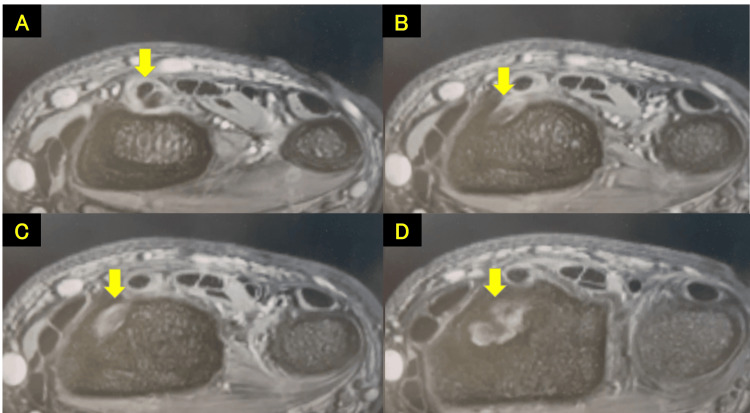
Magnetic resonance imaging scans showing EDC entrapment within the medullary cavity of the radius (yellow arrow) From A to D, cranial to caudal. EDC, extensor digitorum communis
